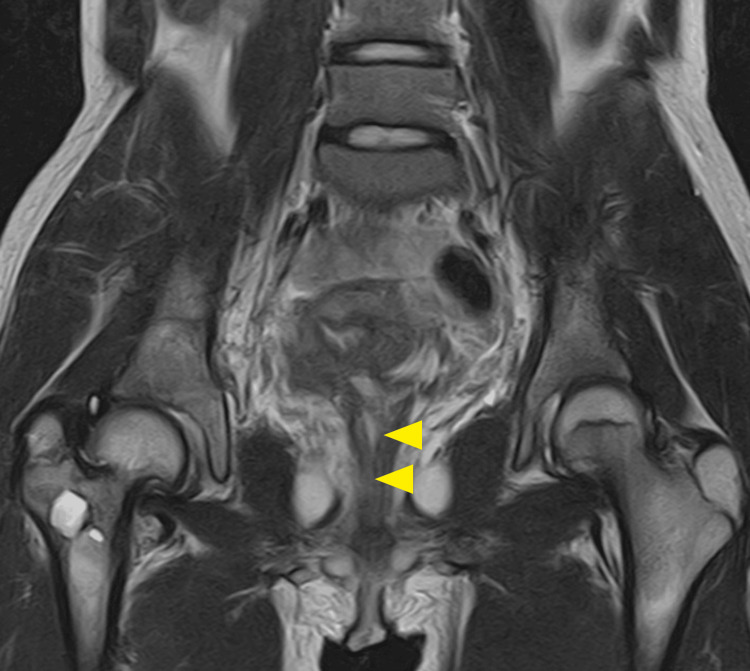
Coronal MRI image at approximately three months postoperatively. No fluid collection or effusion was identified within the reconstructed vaginal canal.
